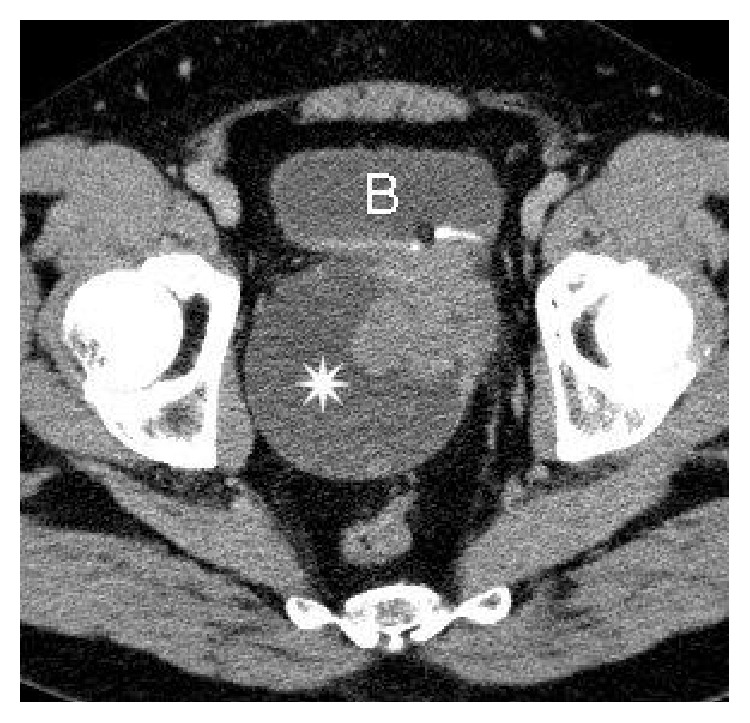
CT of the pelvis, enhanced axial image. The asterisk is on the cystic component of the mass. The arrow shows the solid part of the lesion. B = bladder.
